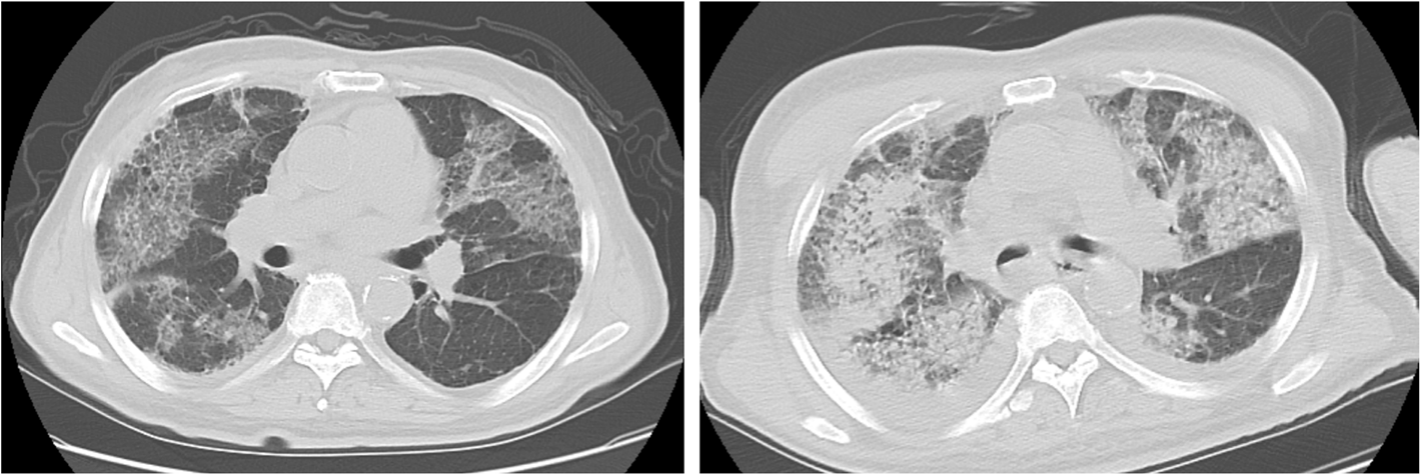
Lung computed tomography at hospital days 1 (Left Panel) and 4 (right panel). Although these findings strongly suggested COVID-19, both of RT-PCR examinations resulted negative
